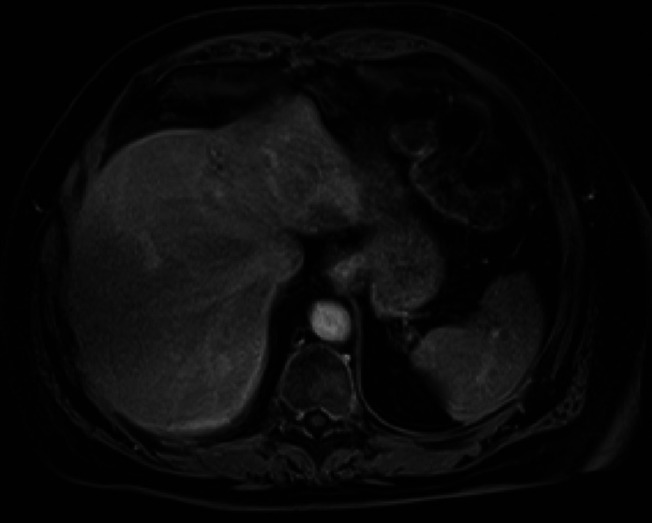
T1-weighted imaging of the liver posttreatment of *M. fortuitum.*
